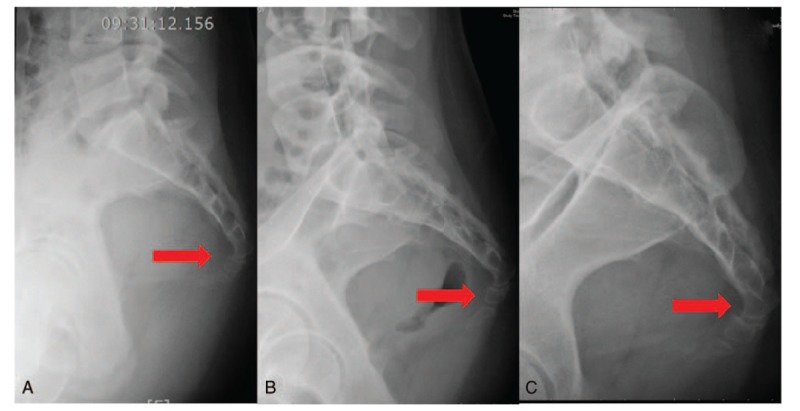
Lateral pelvic radiograph of the fracture at onset and post-therapy. (A) Image acquired immediately after the accident, which occurred on August 14, 2016. The lateral view of the sacrococcygeal joint shows joint deformity after trauma (arrow). (B) Lateral pelvic radiograph acquired after 11 weeks of treatment. This film was taken on March 1, 2017, at which point the clinical symptoms and numerical rating scale score had improved significantly. Bone healing was progressing without further dislocation (arrow). (C) Lateral pelvic radiograph acquired after 24 weeks of treatment. This image was obtained on May 31, 2017, and at this point, the patient was able to perform her daily activities well, without coccydynia.
